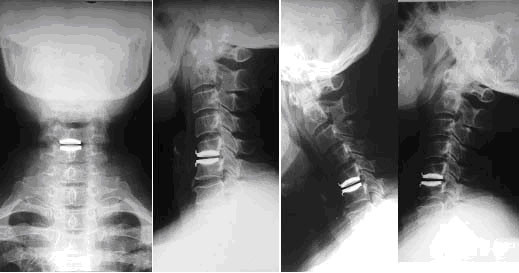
24 months postoperative anteroposterior (A) and lateral (B) x-rays after disc replaced at C5/6, Lateral flexion/extension x-rays(C, D) show restored ROM, and no prosthesis excusion or subsidence.
